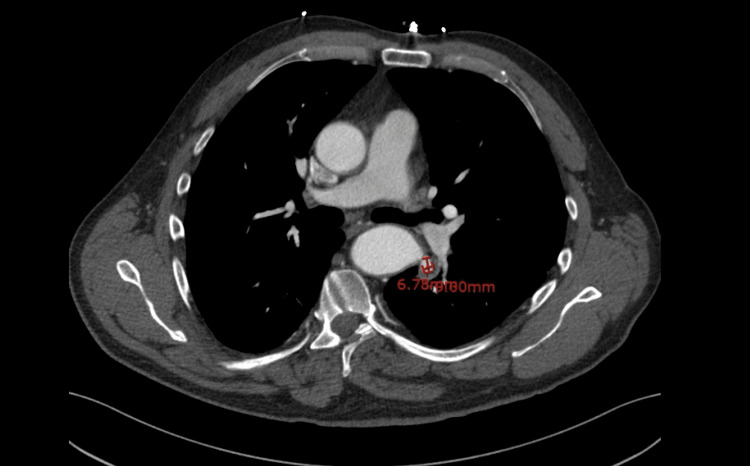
CT angiogram of thoracic aorta showing 10 x 9 mm pseudoaneurysm projecting laterally from the thoracic aorta at the level of previous coarctation repair
